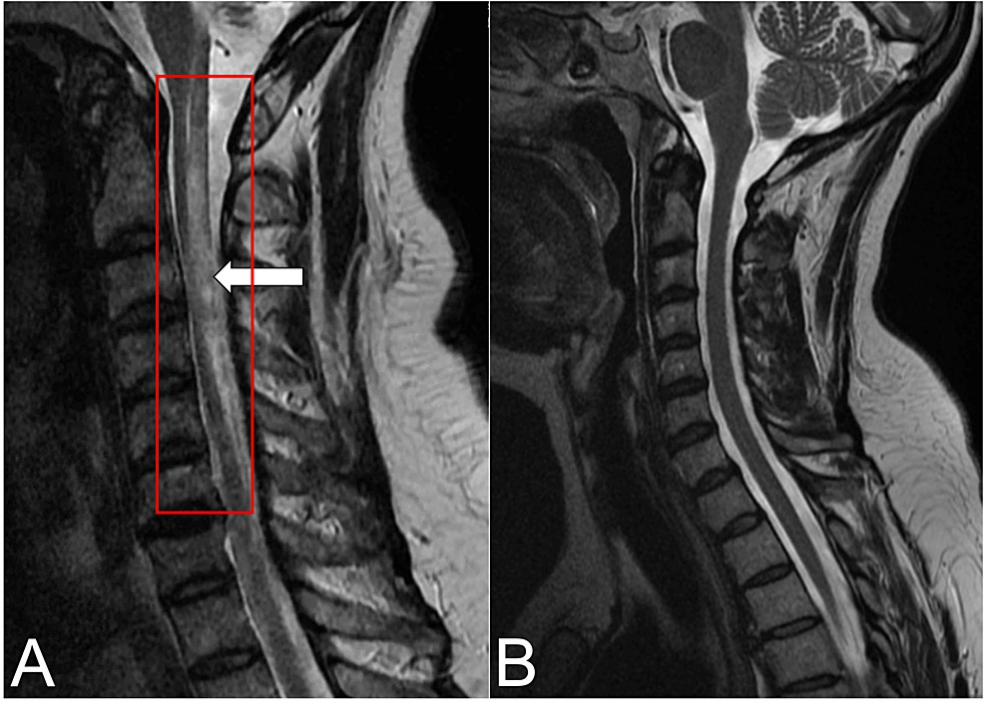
Sagittal view C-spine MRI Sagittal view T2-weighted C-spine MRI of patient HF demonstrates a diffuse increased signal intensity throughout the cervical spine cord (red box), representing significant cord edema, myelomalacia, and potential cord contusion (white arrow) (A). For comparison, a normal T2-weighted sagittal view C-spine MRI is provided from a separate 55-year-old patient (B) [7,8]. MRI: magnetic resonance imaging, C-spine: cervical spine
